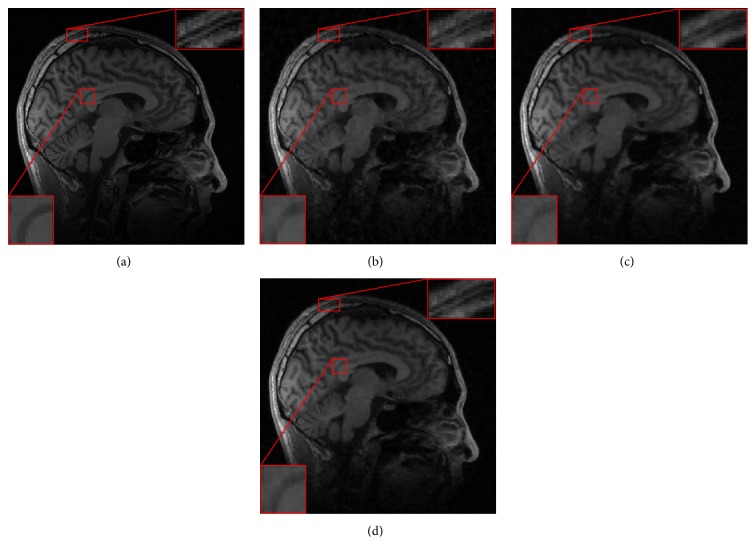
(a) Reference. Reconstructed images from the random sampling mask with accelerating factor *R* = 3 by (b) CSMRI-TV, (c) DLMRI, and (d) our proposed DDTF-MRI, respectively.
